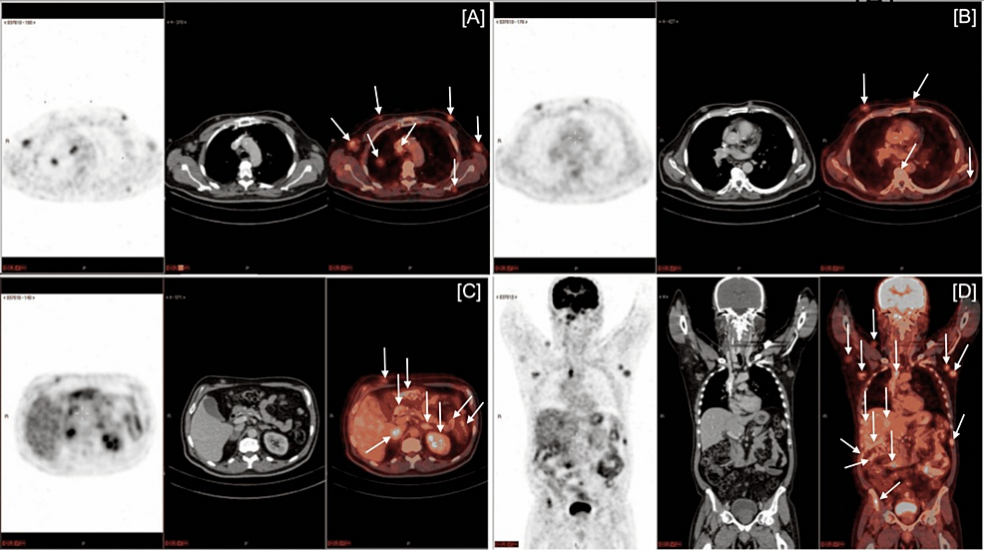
Positron emission computed tomography (PET-CT) scan images. Explanatory Note: Arrows indicate the most prominent uptake areas on PET-CT.
